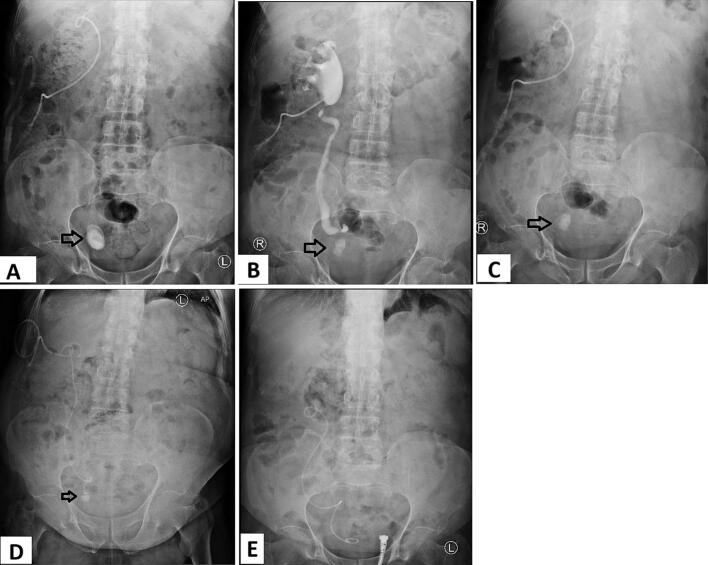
Chronologically ordered KUB X-rays. (A) After the first ureteroscopy and laser lithotripsy and showing right nephrostomy placed. (B) Antegrade nephrostogram showing a significantly dilated right pelvicalyceal system and right ureter with the distal ureter obstructed by the stone. (C) After the second ureteroscopy and laser lithotripsy, (D) showing right nephrostomy and antegradely inserted DJ stent in place, with the tip of the stent abutting the residual stone fragment. (E) Final image after complete fragmentation and clearance of the stone and fragments, and its shows the right DJ stent in place, being distally in the urinary bladder, and proximally in the dilated upper ureter. Arrow pointing to the right distal ureteral stone.
